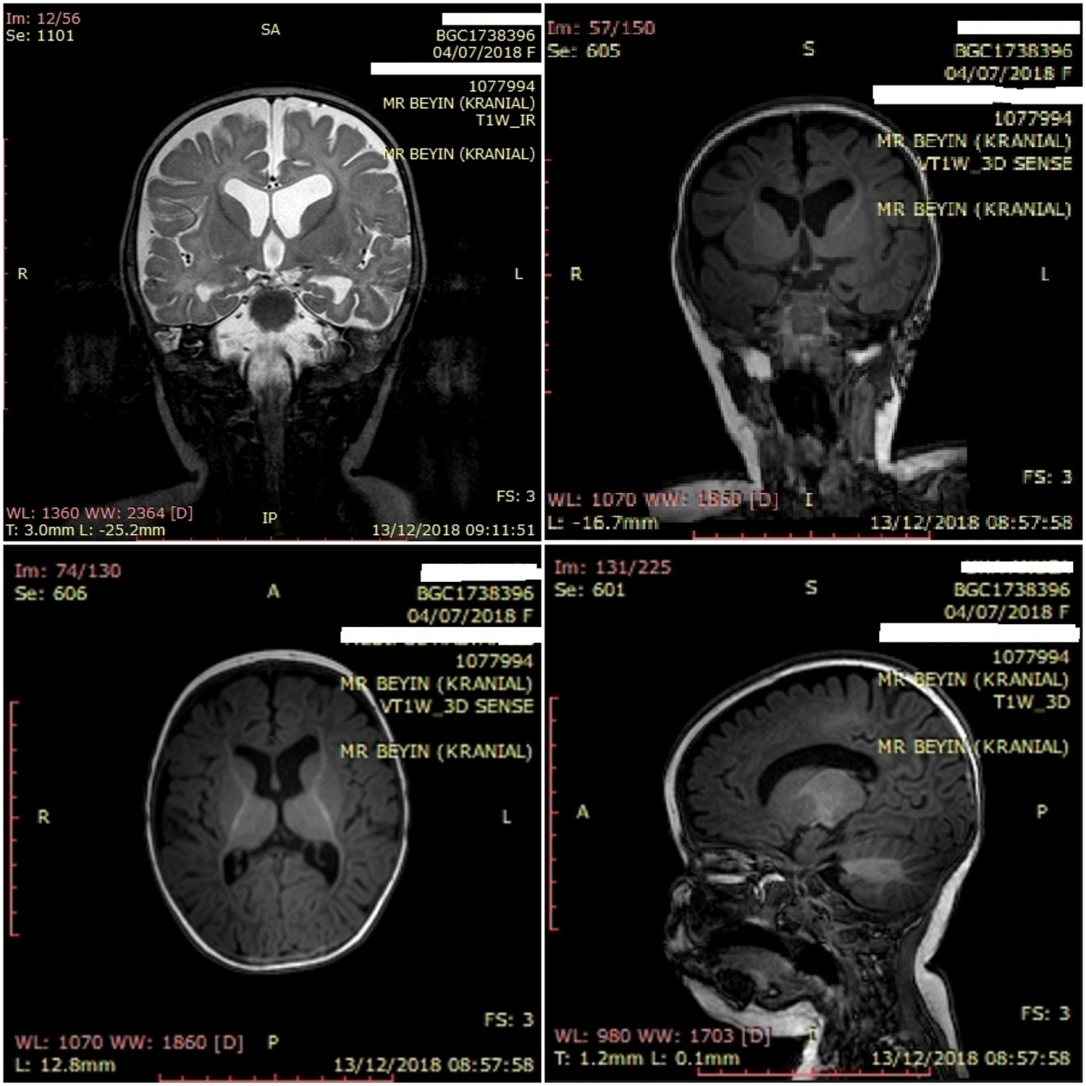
Brain magnetic resonance imaging (MRI) of the patient.
